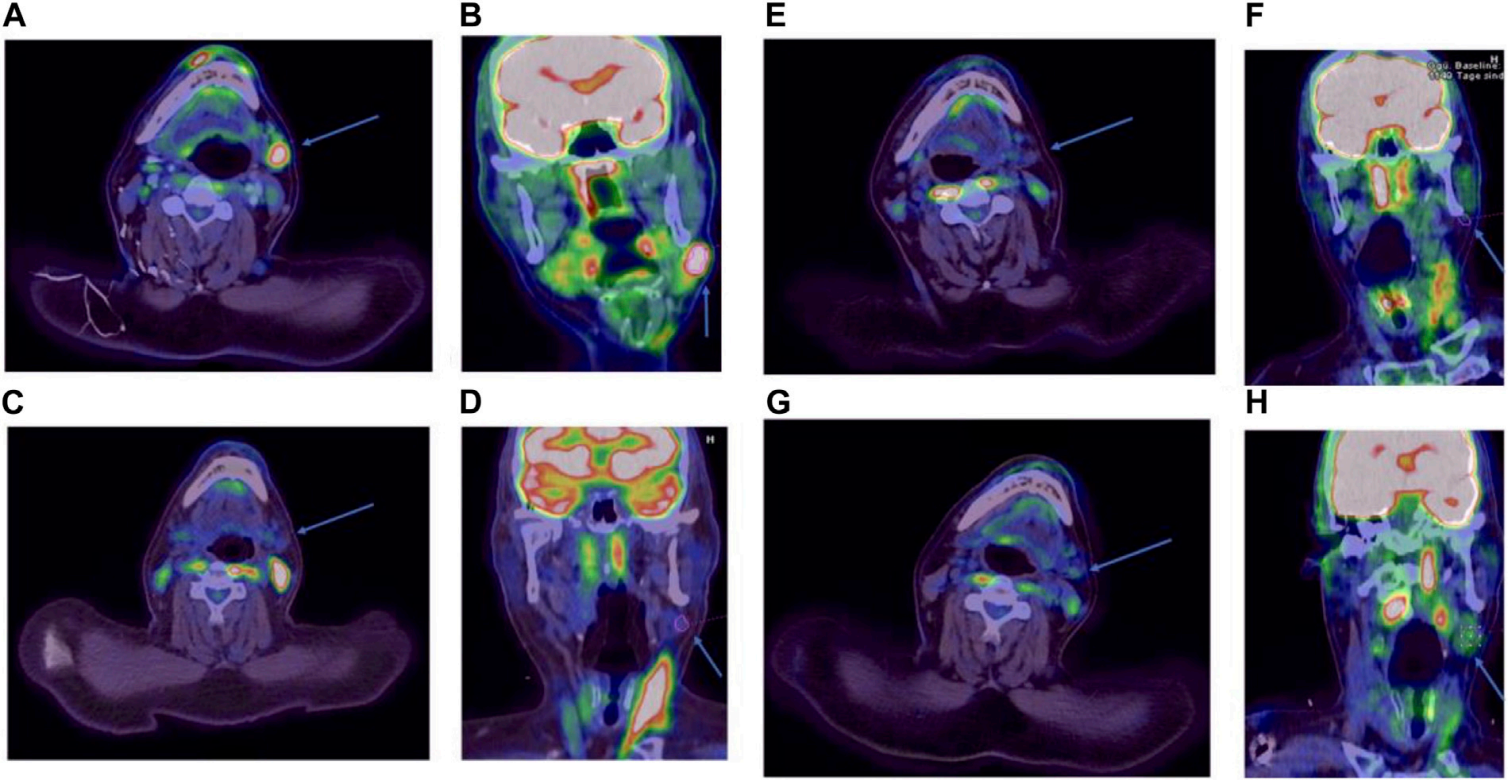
PET/CT images in axial and coronal reconstruction. The target lesion is indicated by an arrow. FDG avidity is expressed by the Deauville score (DS). Before initiation of MEPED, DS: 4 (A, B). After 3 months of therapy, DS: 3 (C, D). After 9 months of therapy, DS: 3 (E, F). Two months after discontinuation of therapy, DS: 3 (G, H).
